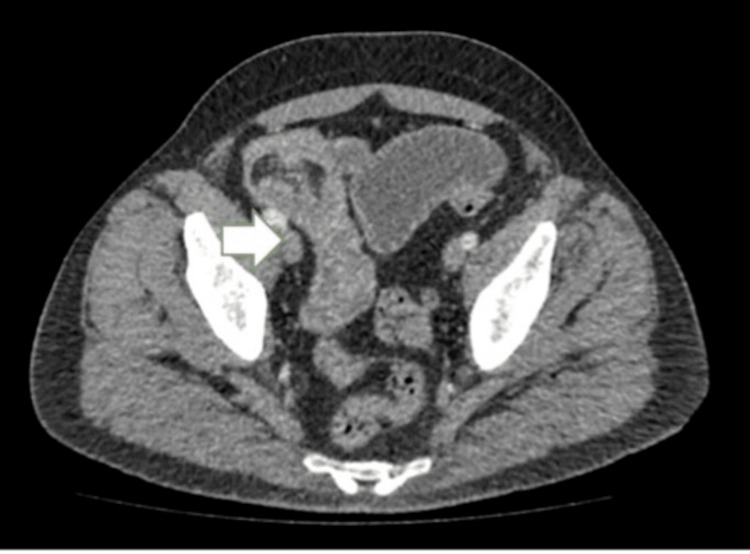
Contrast-enhanced CT abdomen and pelvis (axial section) showing ileum obstruction (white arrow)
